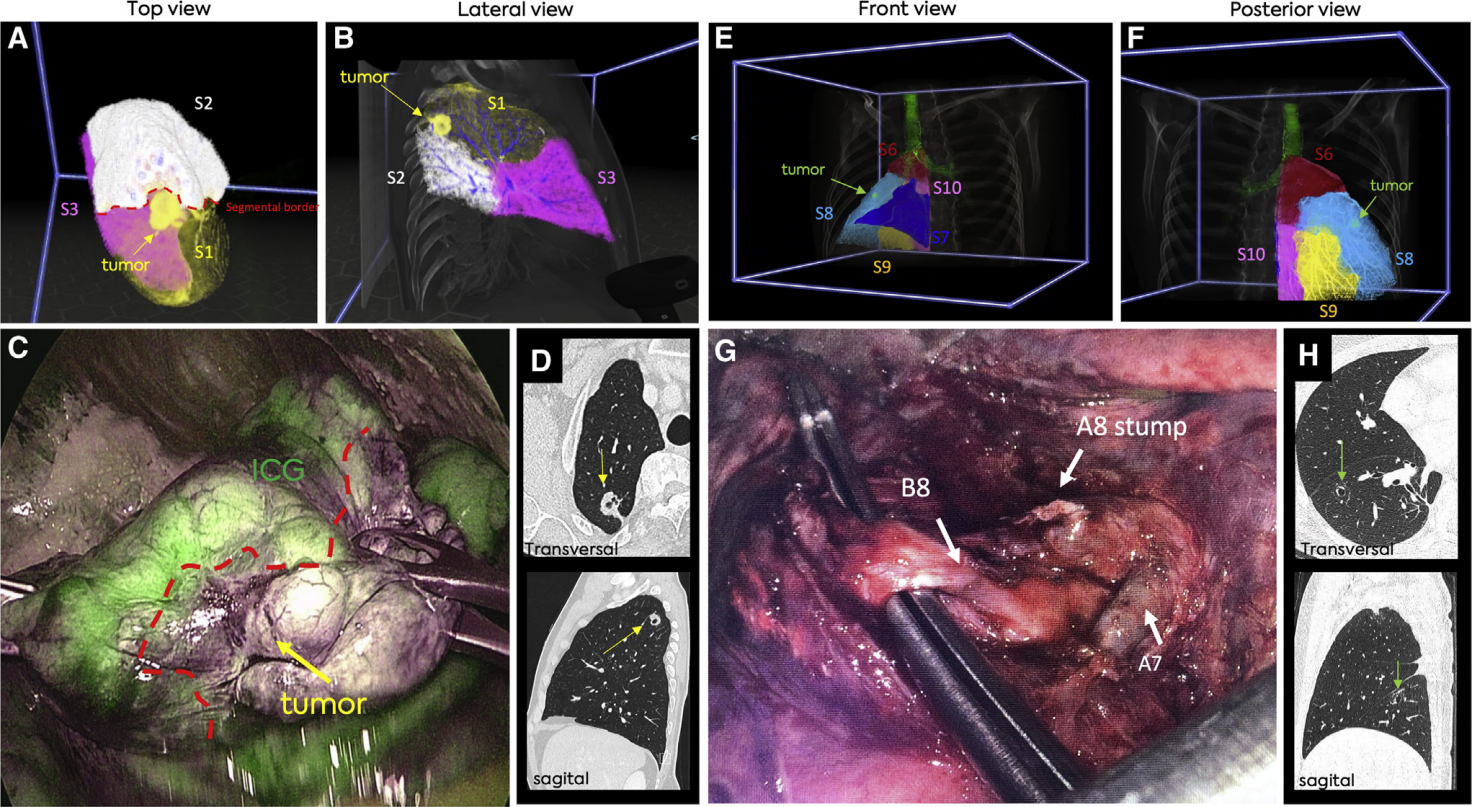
Two representative cases of virtual reality (VR)-adjusted segmentectomy planning. A and B, Two snapshots of the VR-rendered images in a patient with a tumor in segment (*S*) 1. The tumor (*yellow arrow*) and its small margins to S2 are visualized (*red dotted line*). C, Intraoperative screenshot of video-assisted thoracoscopic surgery (VATS) during indocyanine green (*ICG*)-based demarcation of the segmental border (*red dotted line*) between S1 and S2, showing correspondence with the VR images in A and B. D, Representative snapshots of transversal and sagittal computed tomography scan of patient 4. E and F, Two snapshots of patient 7 with a tumor (*green arrow*) in S8. The close relation of the tumor with the lower part of S6 can be seen in panel F. G, Intraoperative screenshot of VATS after ligation of segmental artery (A) 8. H, Representative snapshots of transversal and sagittal computed tomography scan of patient 7. *B8*, Segmental bronchus 8.
